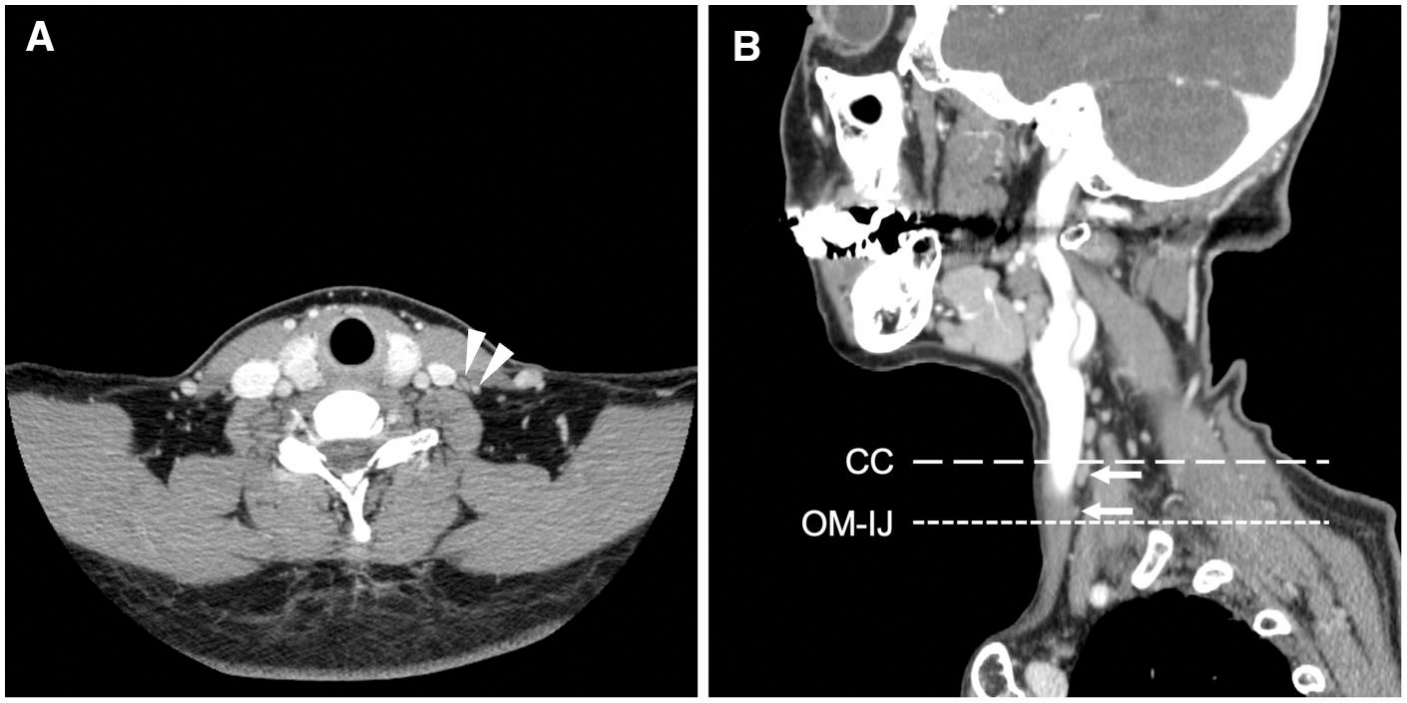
Example of axial and sagittal image of contrast-enhanced CT image with soft tissue windows. (A) The cervical lymph nodes in level III were observed (white arrowheads). (B) The cervical lymph nodes in the gap between the intersection of the omohyoid muscle and the internal jugular vein (OM-IJ) and the inferior border of the cricoid cartilage (CC) were observed (white arrows).
